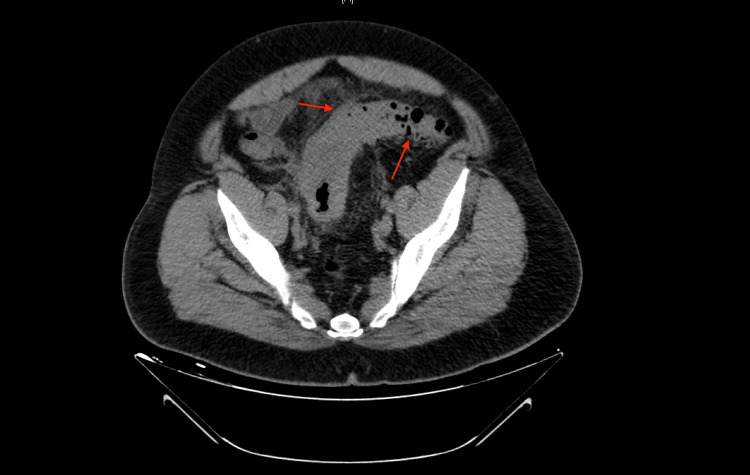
Transverse section of CT abdomen and pelvis without contrast from the initial emergency department visit showing colonic wall thickening and fat stranding (red arrows) consistent with uncomplicated diverticulitis
